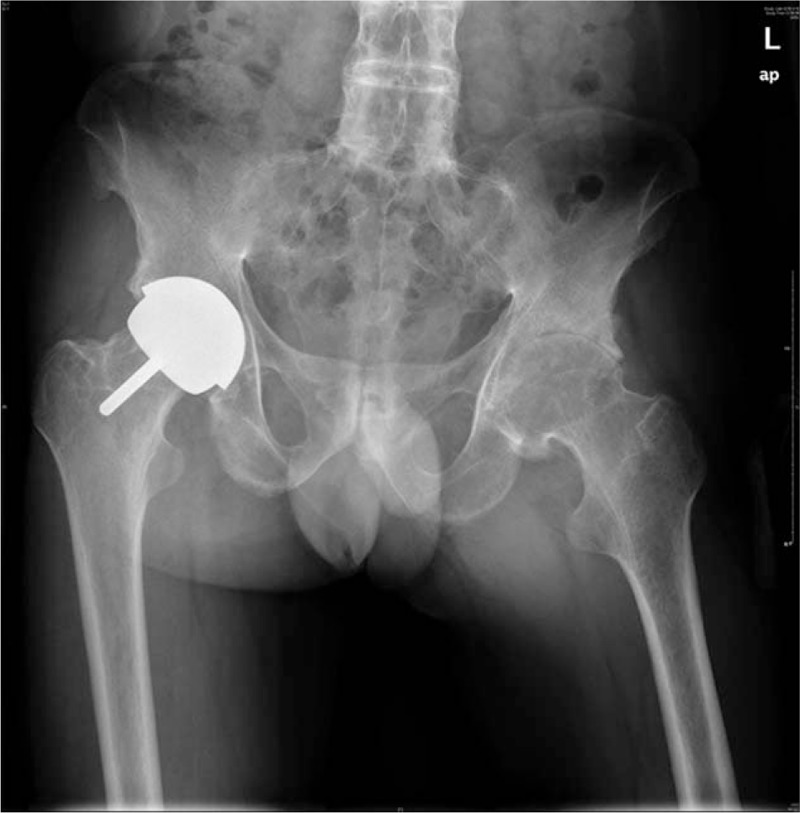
The radiograph of the pelvis of the patient shows bony ankylosis of the lumbar vertebra and bilateral sacroiliac joints. The right hip underwent hip resurfacing 5 years ago, and the left hip was narrowing.
